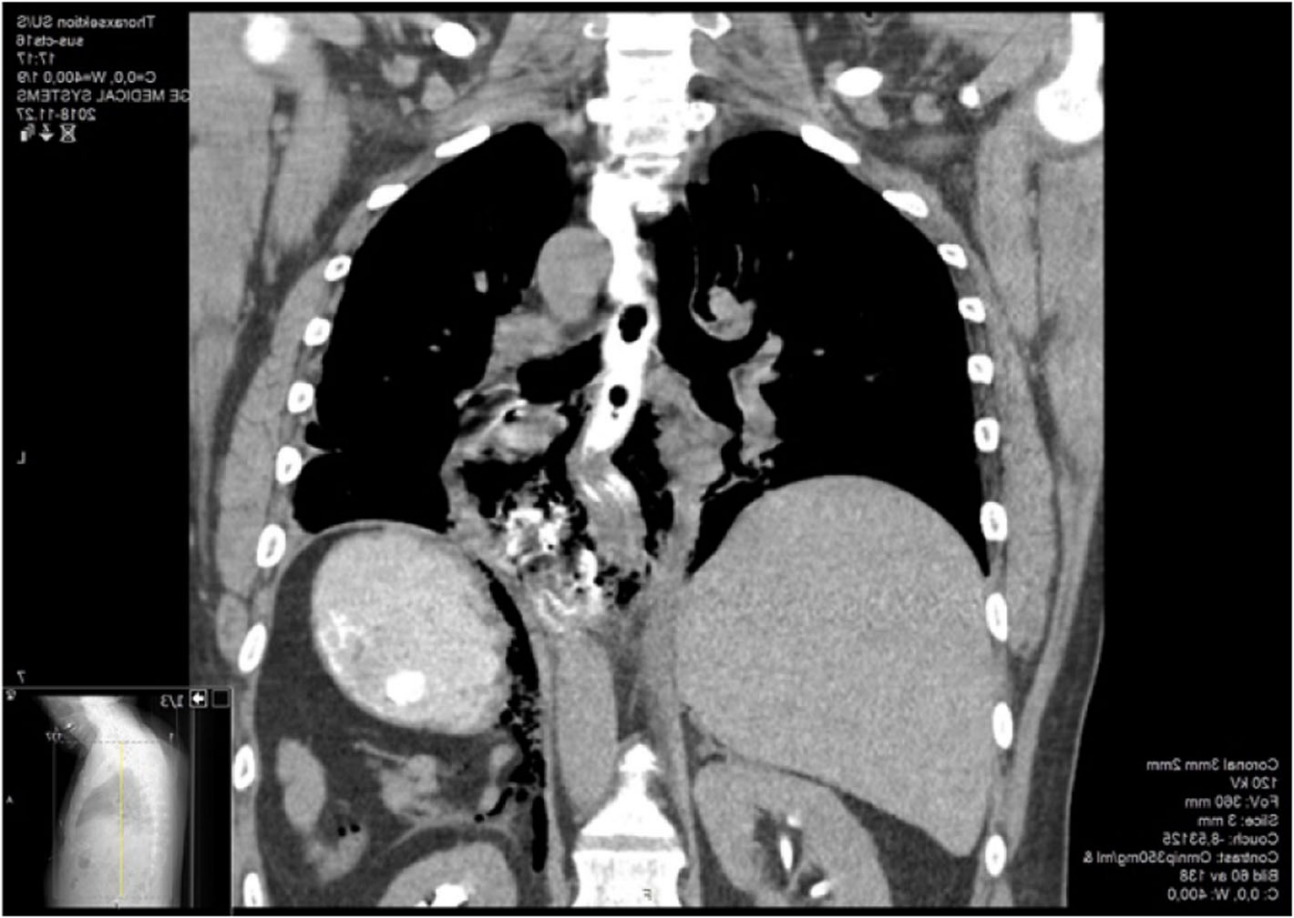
CT image, about 1 h after the injury; demonstrates air in the mediastinum and esophageal rupture
